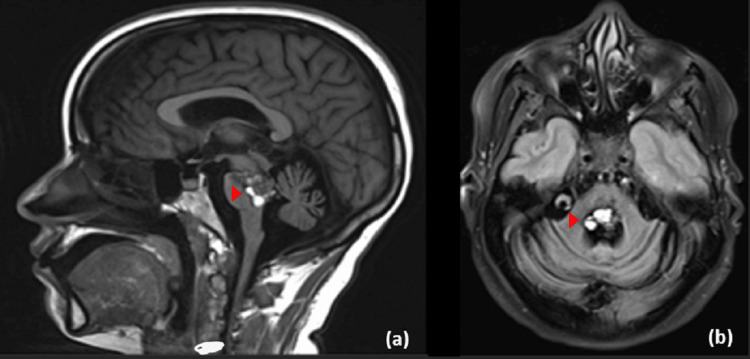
Post-operative magnetic resonance imaging Post-operative sagittal (a) and axial (b) cut of magnetic resonance imaging showing an interval increase in the size of the brainstem cavernoma that now measures 21 x 20 mm but no evidence of new hemorrrhage.
